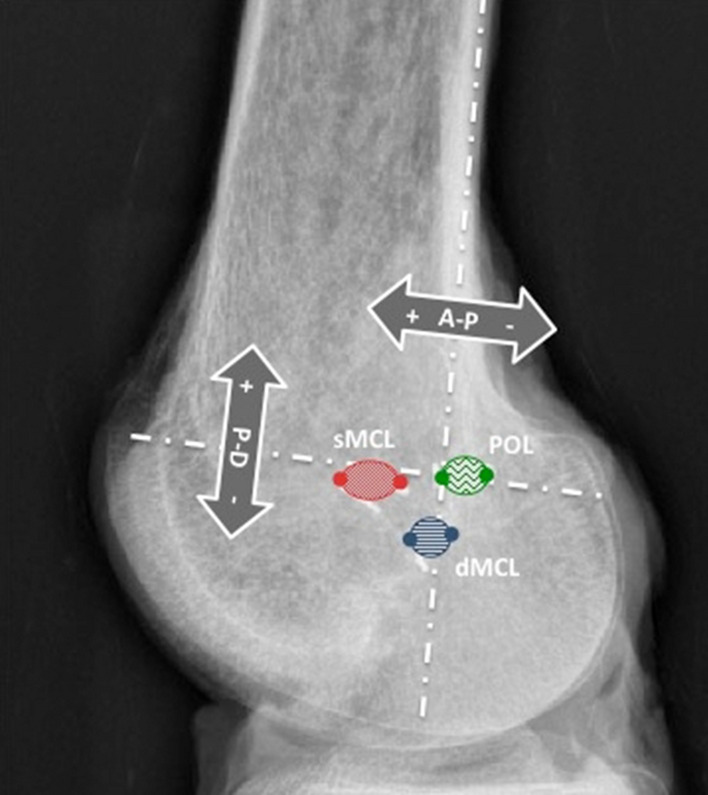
Method to define positions radiographically, using datum lines that (1) form an extension of the line of the posterior femoral cortex, and (2) a line perpendicular to the former passing through the most-posterior/proximal point of Blumensaat’s line [27]
